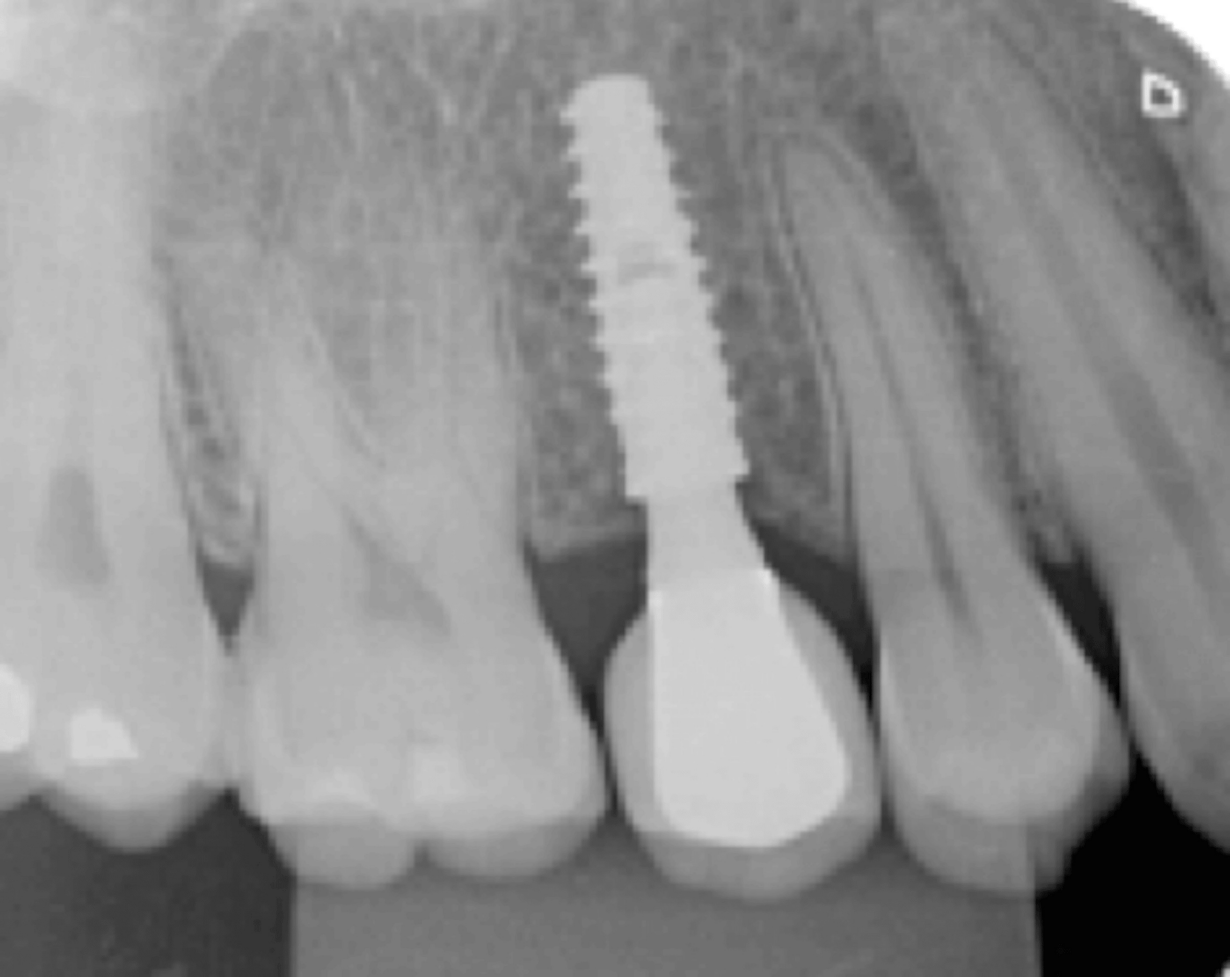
Radiograph showing abutment and provisional restoration installed for implant 15
